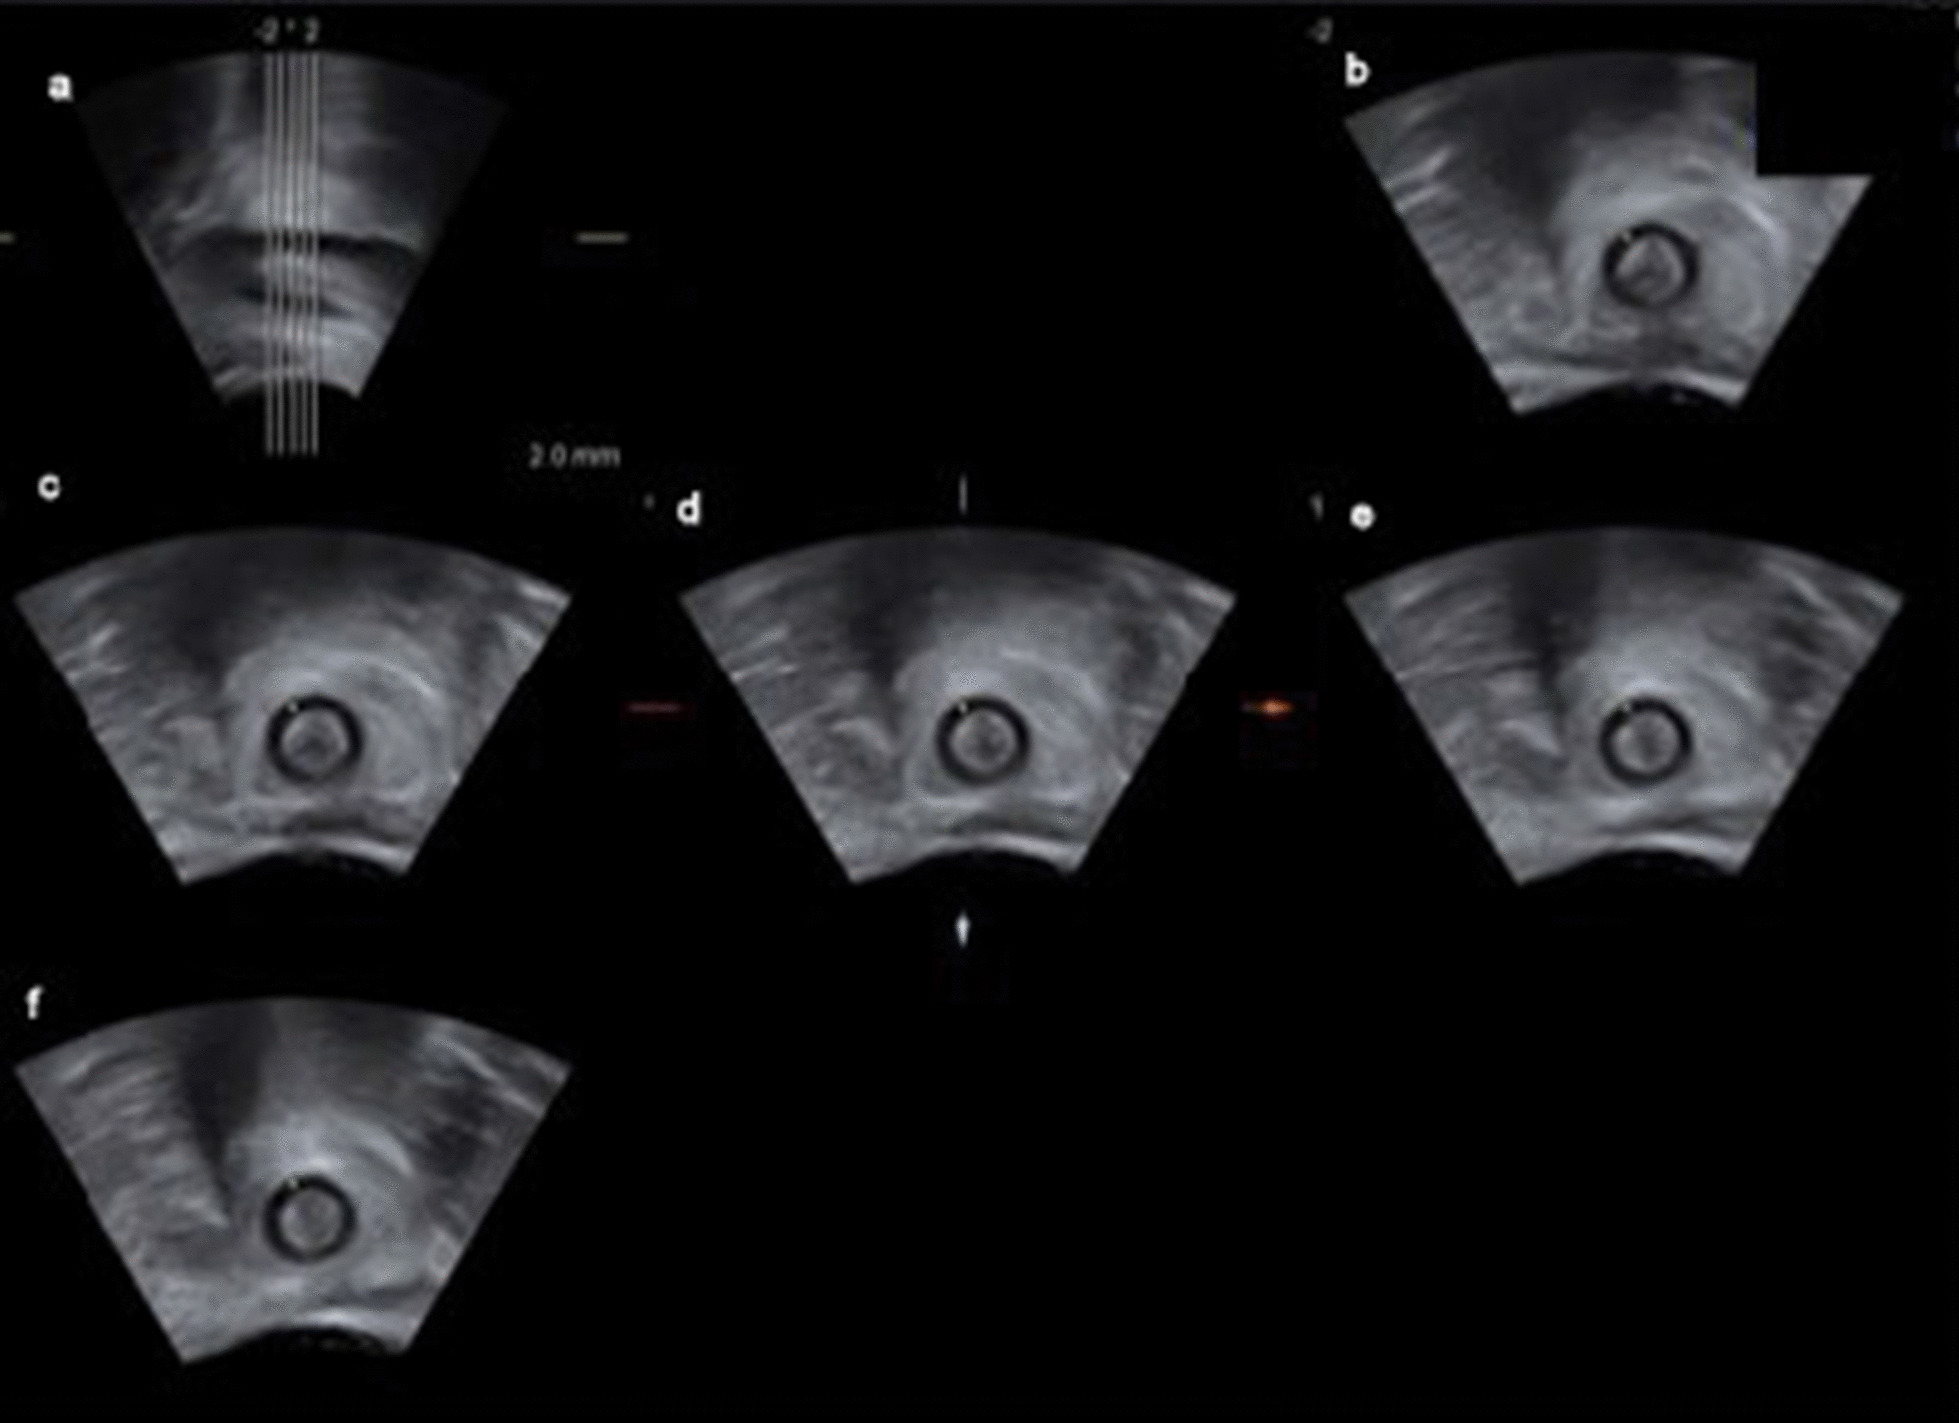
Perianal tomographic ultrasound imaging (TUI) of the anal canal. TUI slices with a width of 2 mm. a Midsaggital plane with marked TUI slices, followed by the 5 slices in coronal plane, beginning with the lowermost slice (b) where external (EAS) and internal sphincter muscles (IAS) have been both visible completely for the first time starting from the anocutaneous transition zone moving in cranial direction. c Slice 2 mm cranial of b. d 2 mm cranial of c, slice where all measurements were done. e 2 mm cranial of d, f 2 mm cranial of e, most cranial slice where EAS and IAS are still both seen completely
